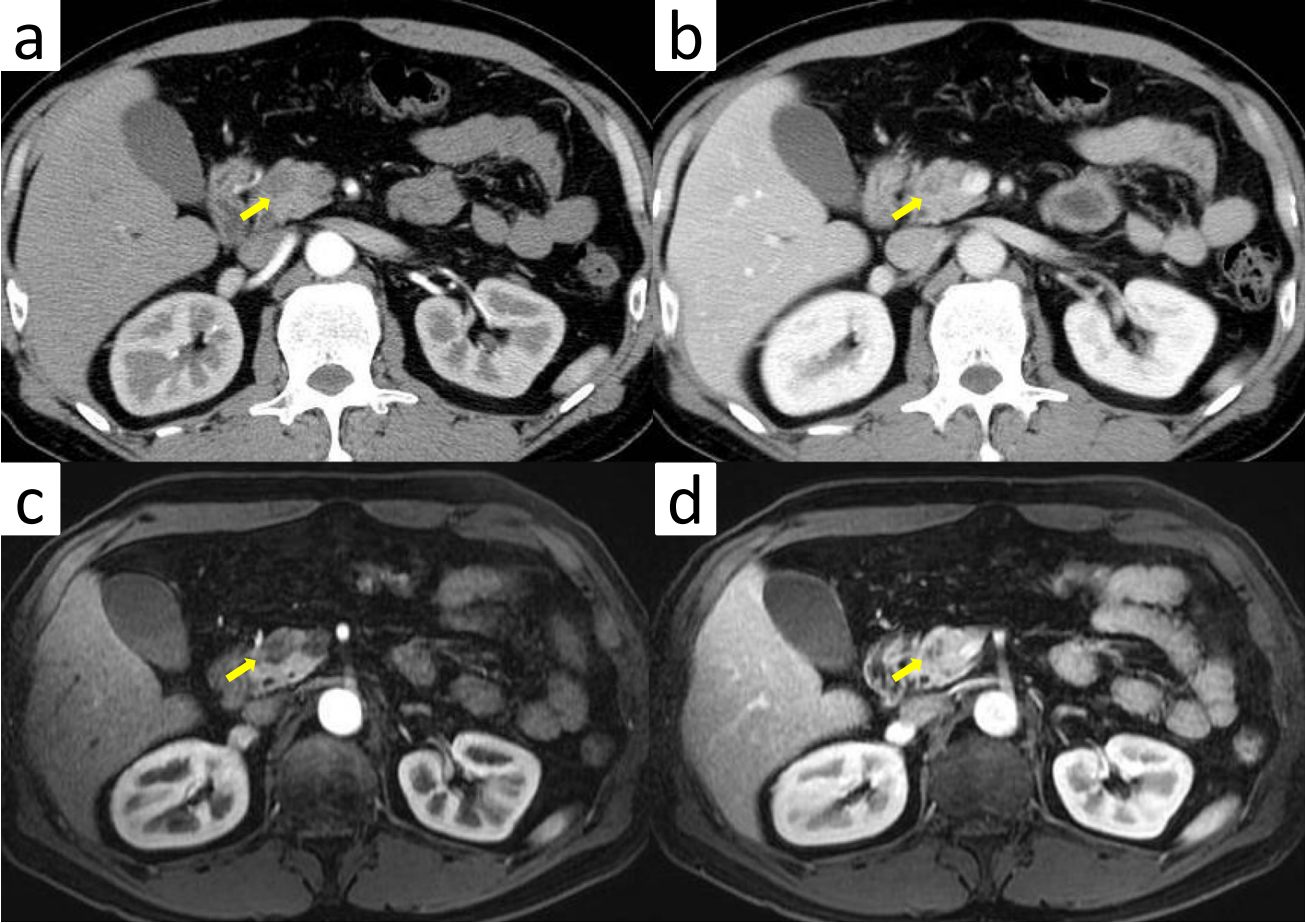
Contrast-enhanced CT and Gd-EOB-DPTA–enhanced MRI revealed a nodule that was gradually contrasted in the pancreatic head (arrow). a Arterial phase of CT. b Portal phase of CT. c Early phase of MRI. d Late phase of MRI. CT, computed tomography; Gd-EOB-DPTA, gadolinium-ethoxybenzyl-diethylenetriamine; MRI, magnetic resonance imaging
